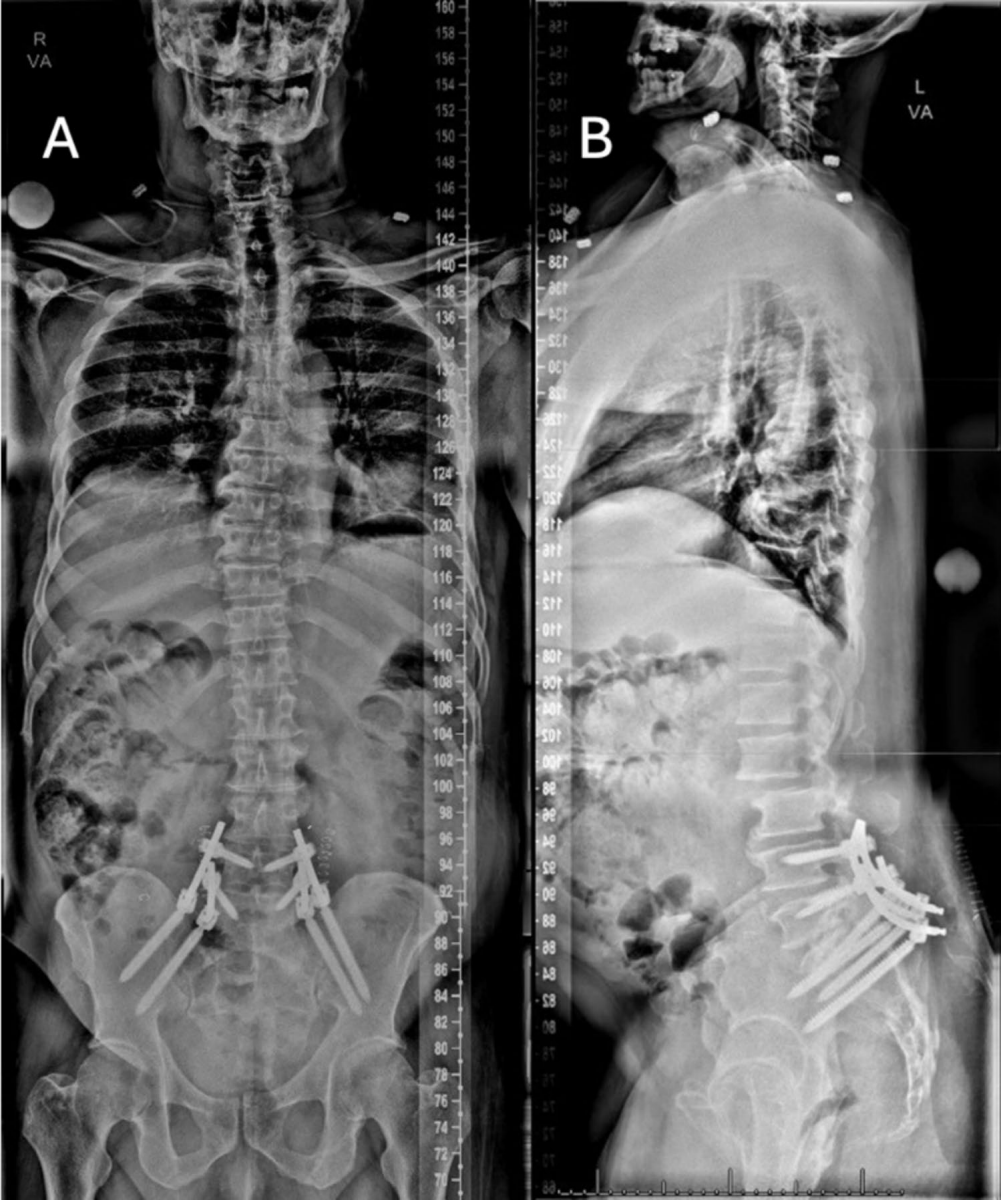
Postoperative upright X-ray. A AP view. B Lateral view (note that this view includes a stitching error between the L2 and L3 vertebral bodies that does not affect visualization of the fusion construct)
